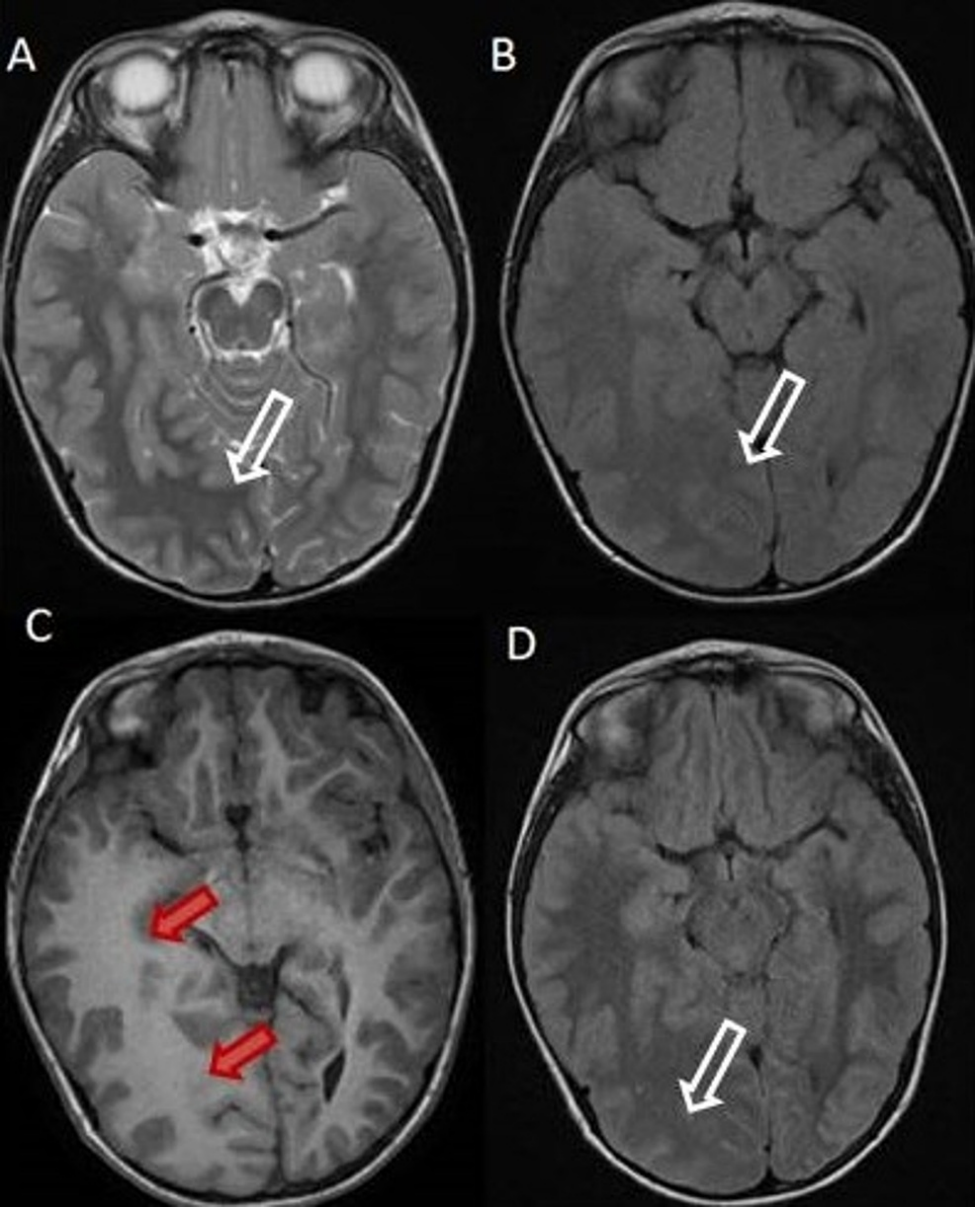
MRI brain, Axial T2 (A), Axial T1 (B), FSGR (C), and FLAIR (D) sequences showing right occipito-parietal cortical dysplasia with relatively bright and bulky white matter (red arrows) and poorly defined cortical ribbon (white arrows).
